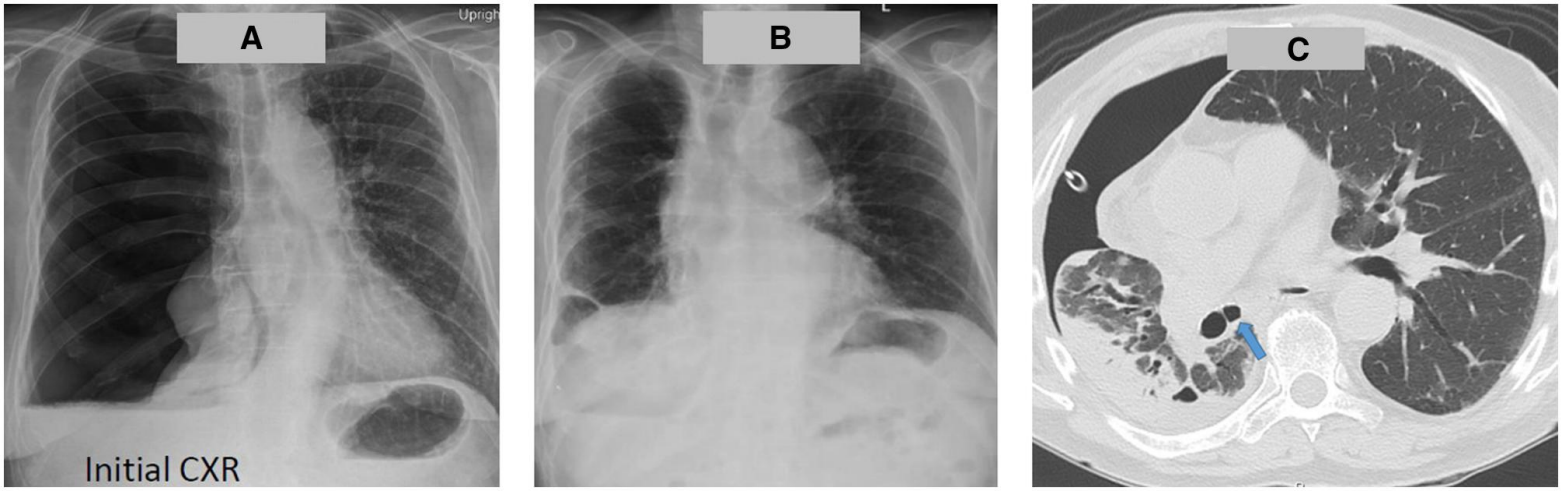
Operative finding and postoperative course: initial CXR (A), 3-month postoperative CXR (B) and bronchial diverticulum on CT chest (C)
